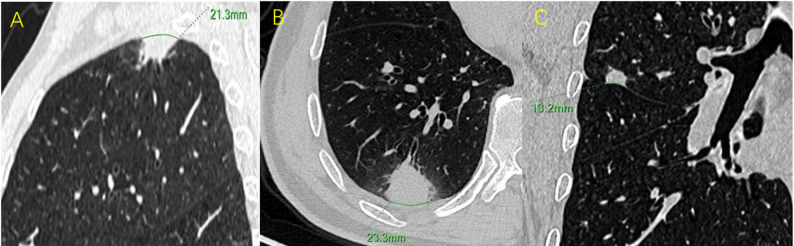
(a–c) Sagittal, axial, and coronal CT images of solid pulmonary adenocarcinoma nodules with pleural contact pathologically confirmed as VPI-positive; preoperative CT shows pleural contact lengths of 21.3 mm, 23.3 mm, and 13.2 mm. Abbreviations: VPI, visceral pleural invasion; CT, computed tomography
